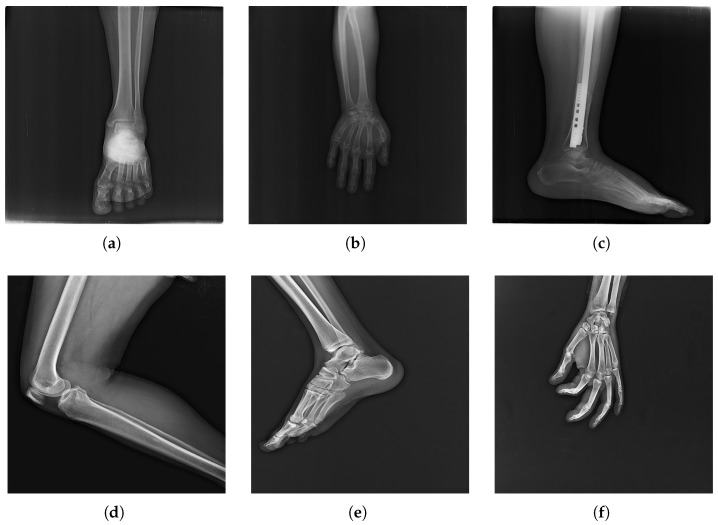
Sample of X-ray images from *FracAtlas* [31], where (a–c) showcase fractures and (d–f) do not contain fractures.
